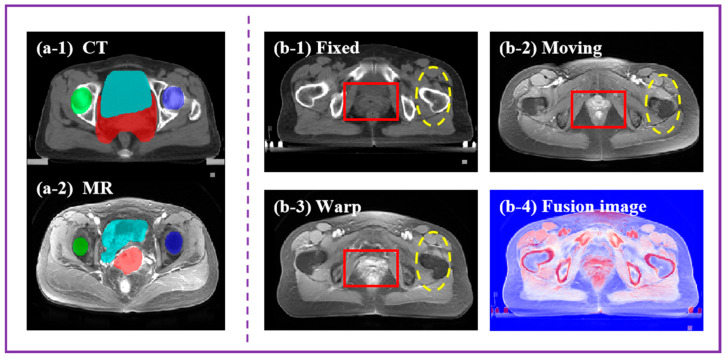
The CT and MR images of the same patient are shown in (a), and the large deformation can be found between the multimodality images, which also increases the difficulty of our algorithm design. (a-1) represents the patient’s CT image, and (a-2) represents the patient’s MR image. (b-1) shows the CT image and (b-2) shows the MR image. (b-3) shows the registration image, and (b-4) shows the superimposed image of the warp image and fixed image. The MTEF model can better learn the features of the fixed image, achieving high-precision multimodality image registration and fusion.
